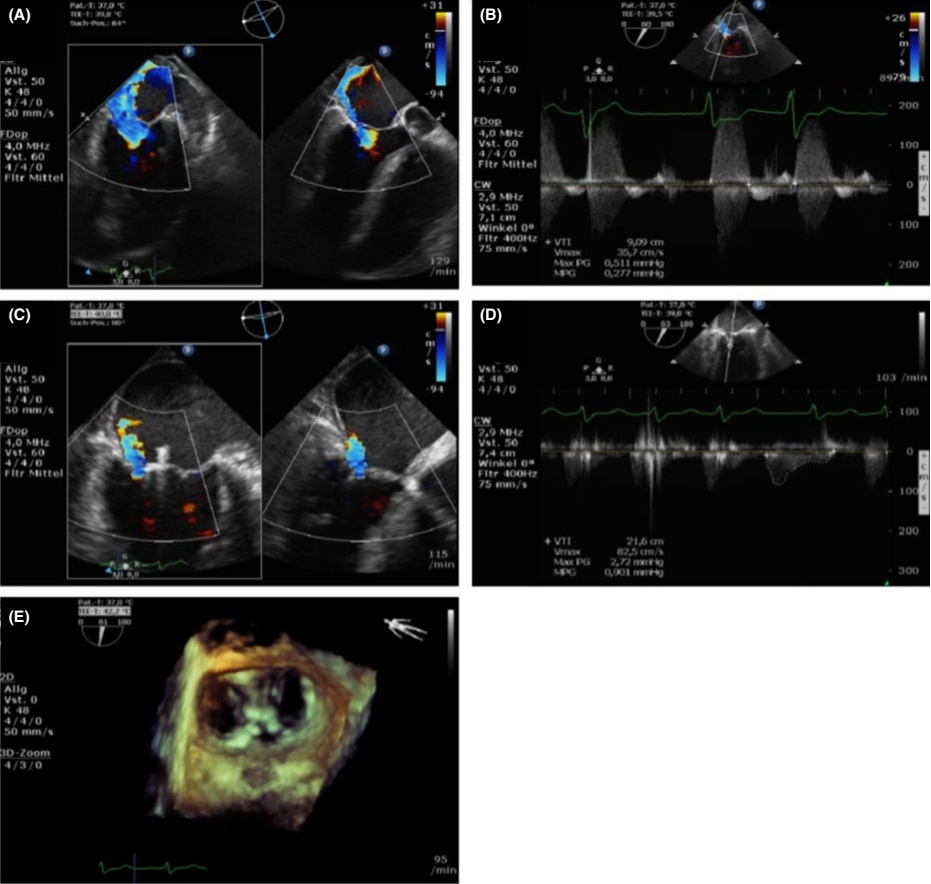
Result of percutaneous mitral valve repair. Comparison of mitral regurgitation before (A) and after (C) clip implantation (transesophageal two‐chamber (left) and left ventricular outflow tract view with color Doppler encoding). Mean transvalvular gradients before (B) and after (D) clip implantation. Surgical view (E) of the mitral valve and the two implanted clips in three‐dimensional reconstruction.
